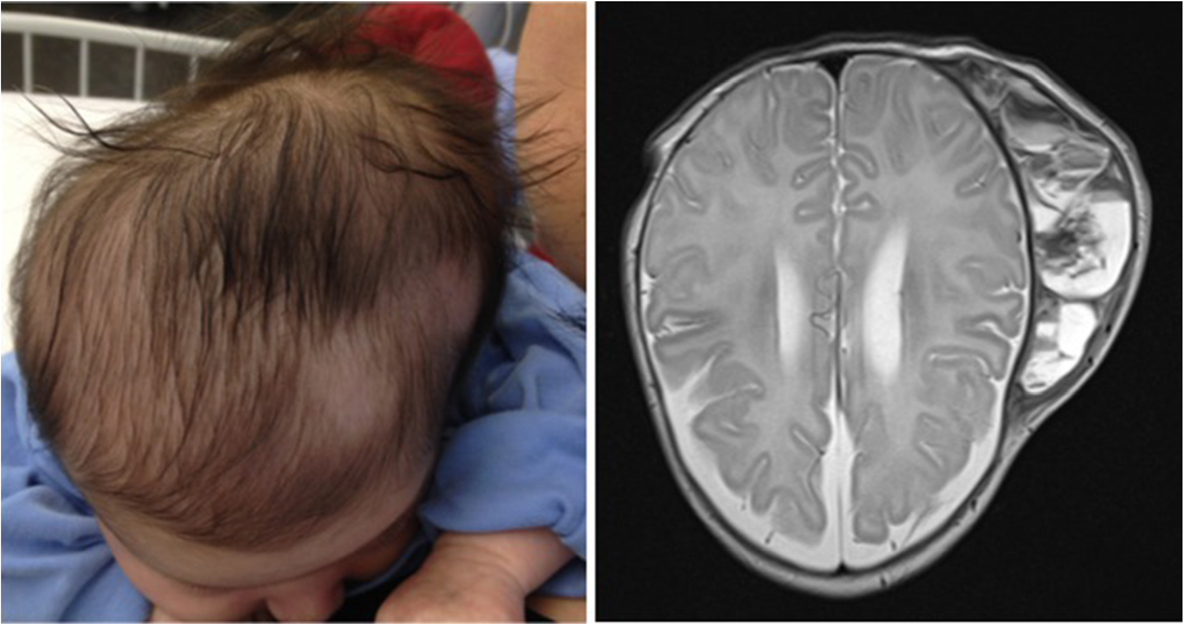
Clinical presentation and magnetic resonance imaging findings of the 5-week-old girl with infected cephalhaematoma
